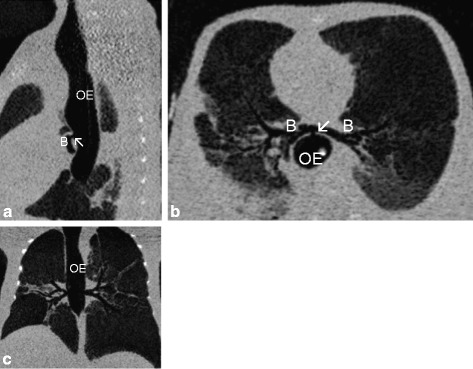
Chest CT scans (sagittal (a), horizontal (b) and axial (c)) show the absence of the trachea and bronchi (B) arising from the oesophagus (OE) via a fistula (arrow ↖)
